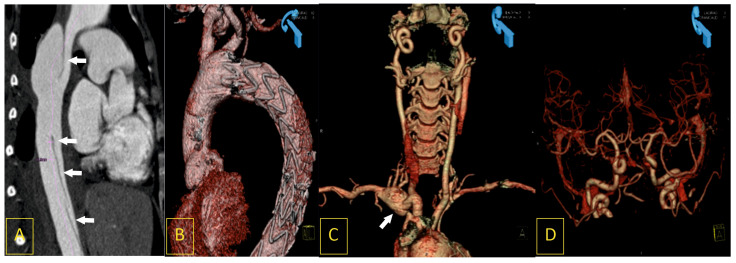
A 28-year-old woman, ten days after the uneventful delivery of a healthy daughter, was admitted to the cardiac emergency unit after a short episode of syncope with severe chest pain and symptoms of deteriorating cardiac shock. Computed tomography angiography (CTA) revealed acute type III dissection of the thoracic aorta (A). It was successfully treated with immediate implantation of endovascular stent-graft prosthesis (B). The same CTA revealed other vascular pathologies that may be found in Ehlers–Danlos syndrome: an aneurysm of the right subclavian artery ((C), white arrow) and arterial loops caused by elongation of the intracranial segments of the internal carotid arteries (D).
